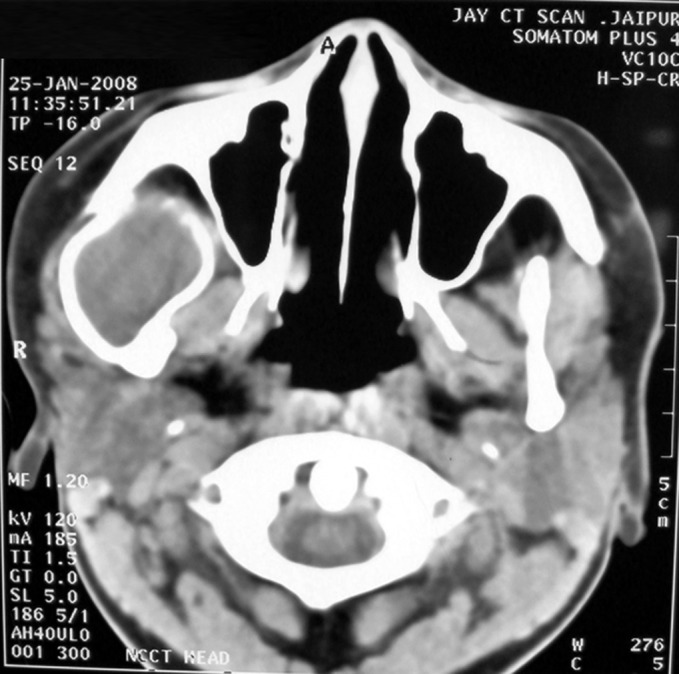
CT scan of secondary lesion showing
